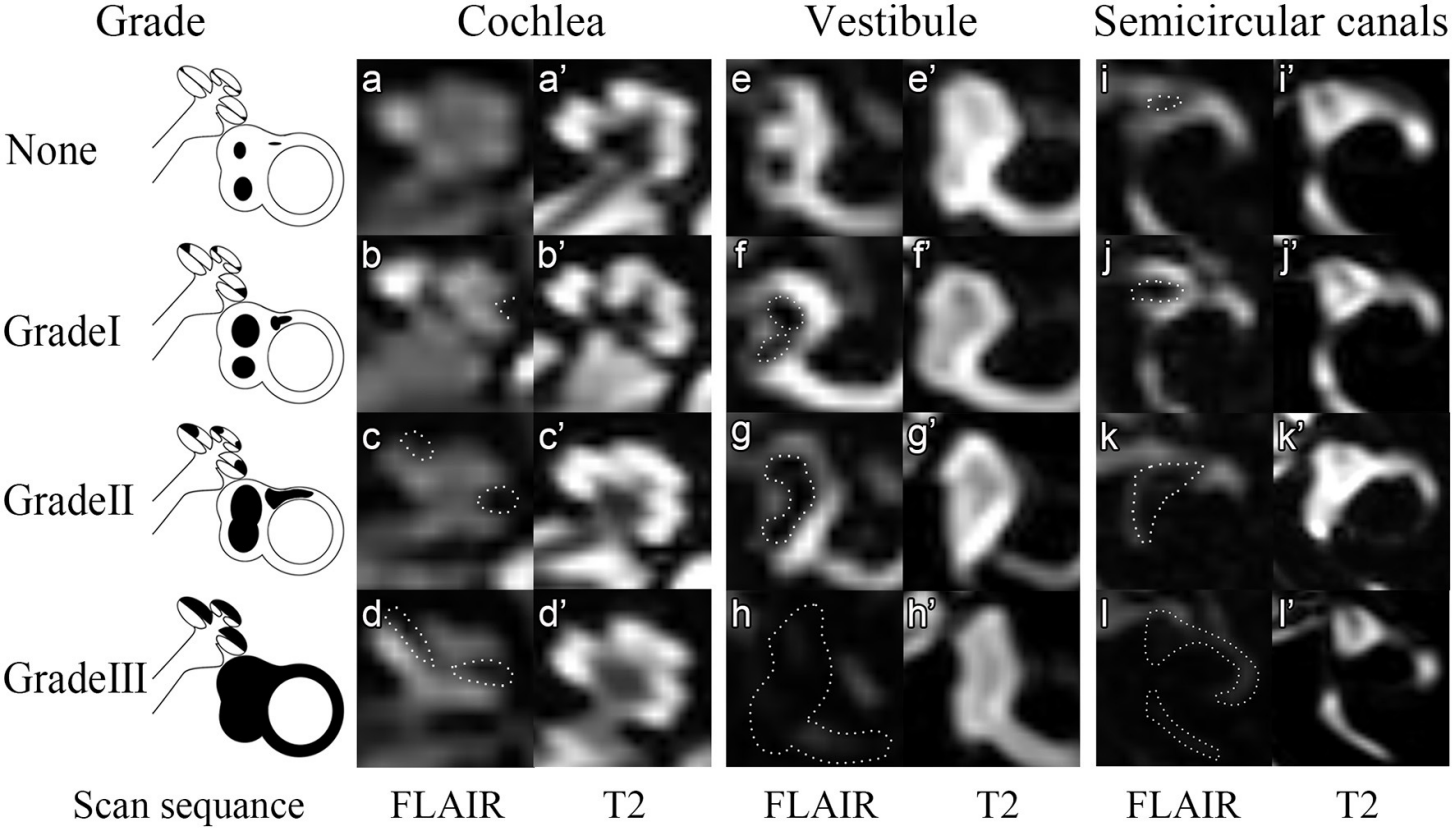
Four grades of the inner ear ELH. In this figure, 3D-FLAIR pictures (a–l) are shown adjacent to T2 weighted image for each grade (a'–l'). None = Normal inner ear. In the normal inner ear, one can recognize no enlargement in the scala media (a,a'). The saccule and utricle are separate and saccule is smaller than the utricle (e,e'). The semicircular canals and a narrow dark area (<1/3 of the ampulla) are visible (i,i'). Grade I = Mild hydrops. In mild hydrops cochlea, an enlarged scala media can be observed with the scala vestibuli around (b,b'). In mild hydrops vestibule, saccule becomes larger than utricle but not yet confluent with utricle (f,f'). In mild hydrops semicircular canals, a dark area occupying over 1/3 of the ampulla is observed (j,j'). Grade II = Moderate hydrops. In moderate hydrops cochlea, the scala media expands into circle (c,c'). In the vestibule, a confluence of the saccule and utricle appears with visible perilymph surrounding (g,g'). In semicircular canals, the crura become dark and some of the canals become invisible. Grade III = Severe hydrops. The scala vestibuli, saccule and utricle and semicircular canals are filled with endolymph so that no signals could be viewed in these areas.
